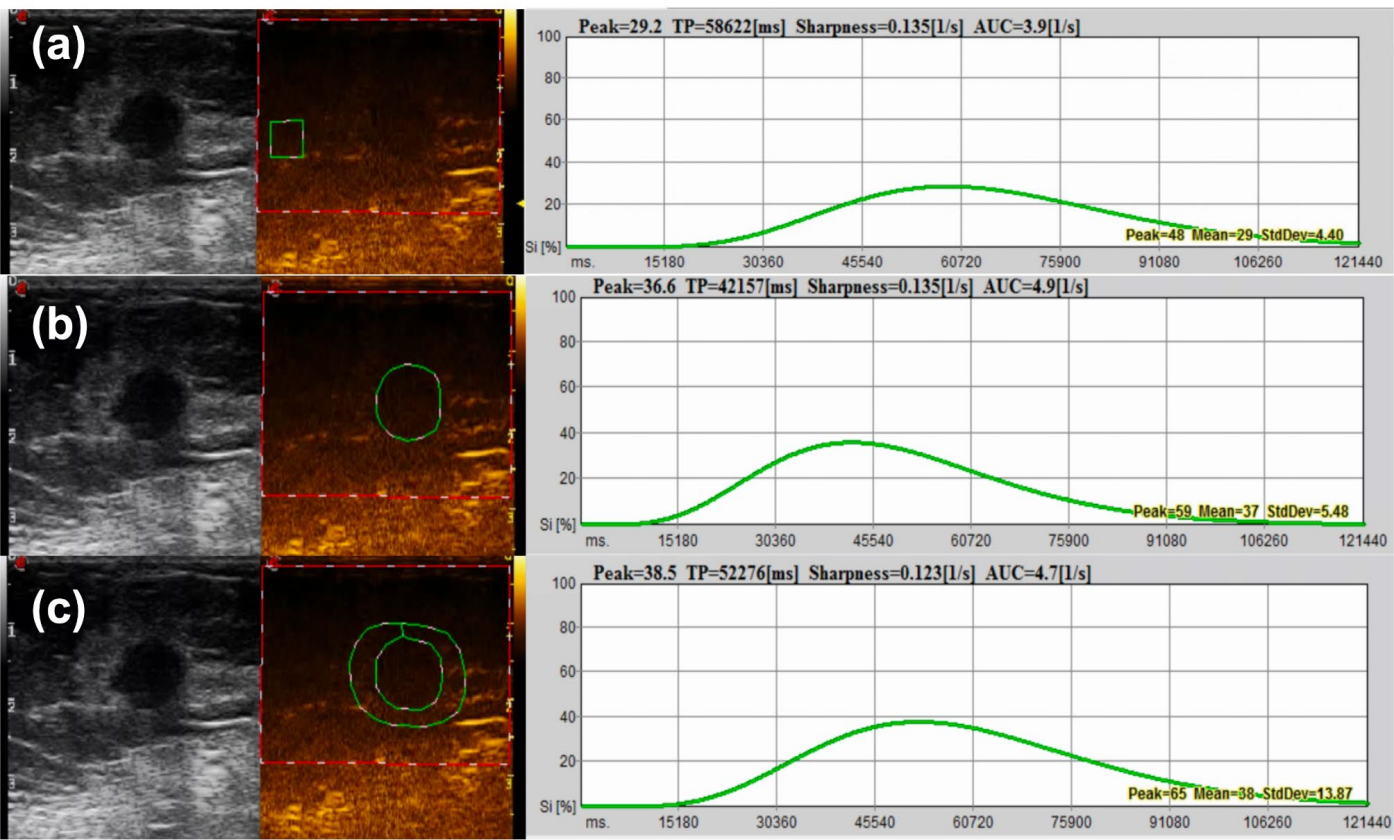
Contrast-enhanced ultrasound (CEUS) time intensity curve (TIC) analysis. The figure showed the Peak and Time to Peak (TTP). (a) ROI1: corpus mamma ROI; (b) ROI2: intratumour ROI; (c) ROI3: peritumour ROI.
